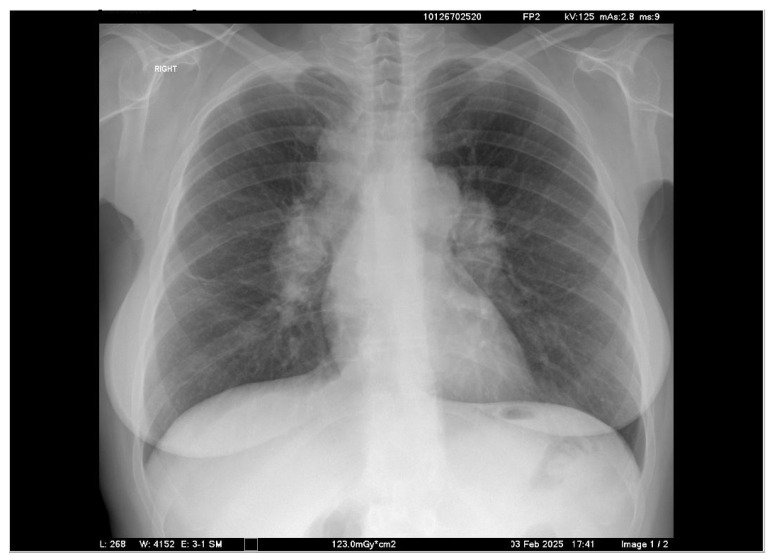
Chest X-ray showing prominent hilar lymphadenopathy.
